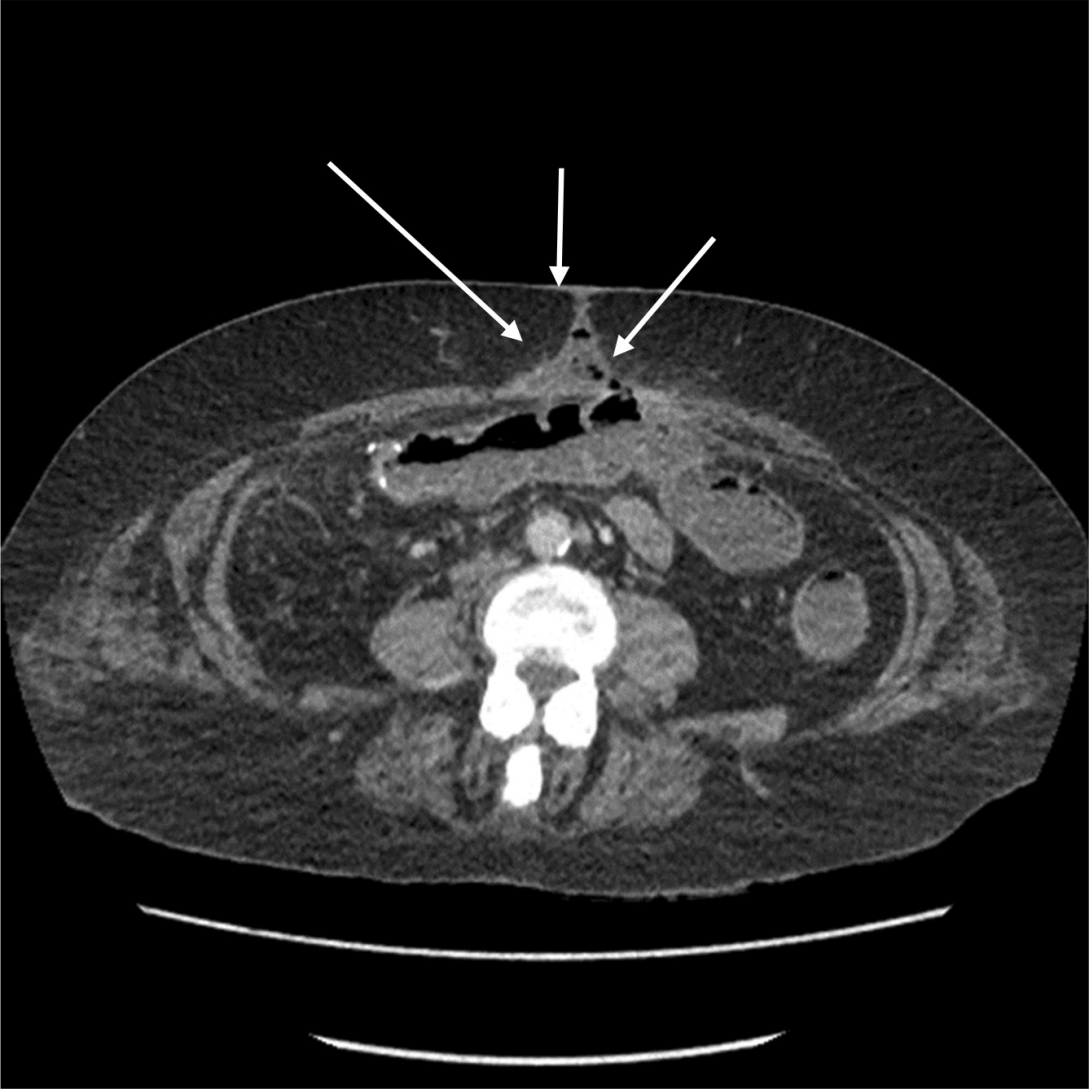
CT scans of the abdomen 20/03/2021; shows New colo‐cutaneous fistula 7.7 cm distal to the anastomosis in the transverse colon.
